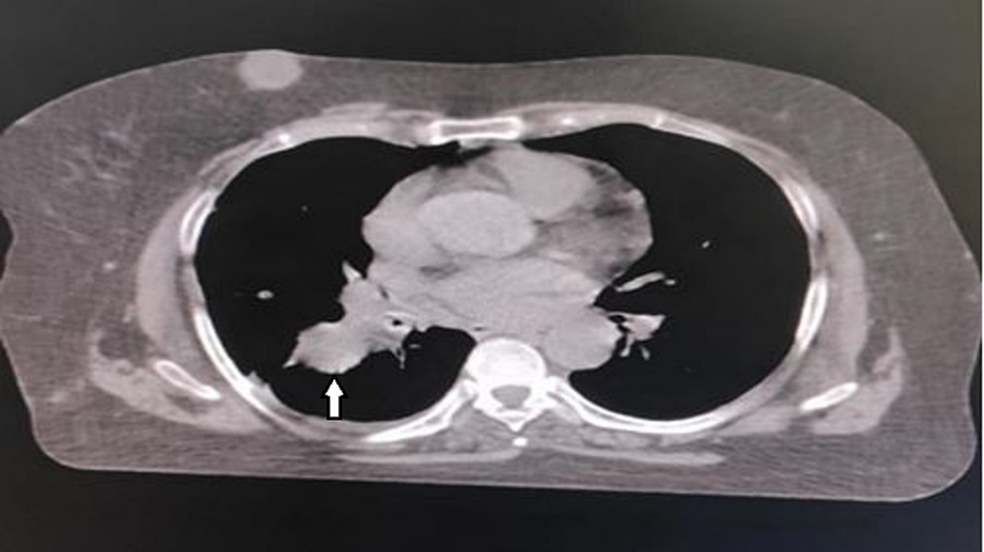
Transaxial CT image of thorax showing mass (marked with white arrow) in the perihilar superior segment of the right lower lobe
